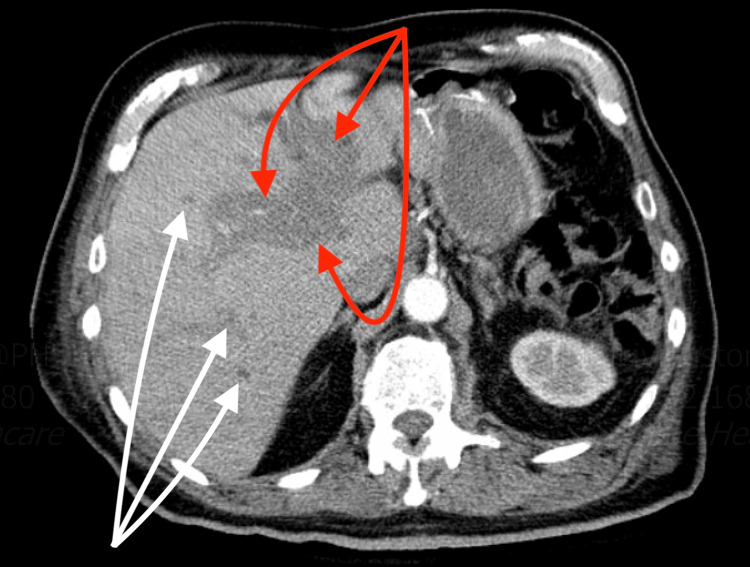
Non-contrast CT scan of the abdomen and pelvis Tumor infiltration (red arrows) of the hepatic peri-portal system of the liver (white arrows). CT: computed tomography
